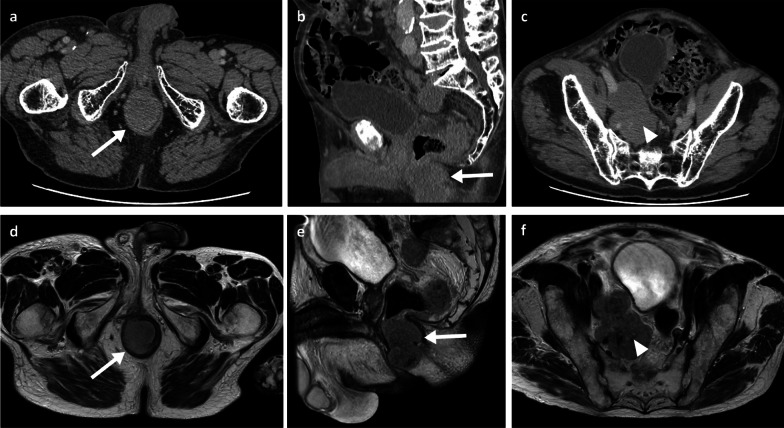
Portal phase contrast-enhanced CT axial (a, c) and sagittal (b), MRI T2WI axial (d, f) and T2WI sagittal (e) of a 77-year-old male patient with a metastatic melanoma anal canal mass (arrows) with extensive lymphadenopathy involving the mesorectal fat, iliac, inguinal and retroperitoneal chains (arrowheads)
